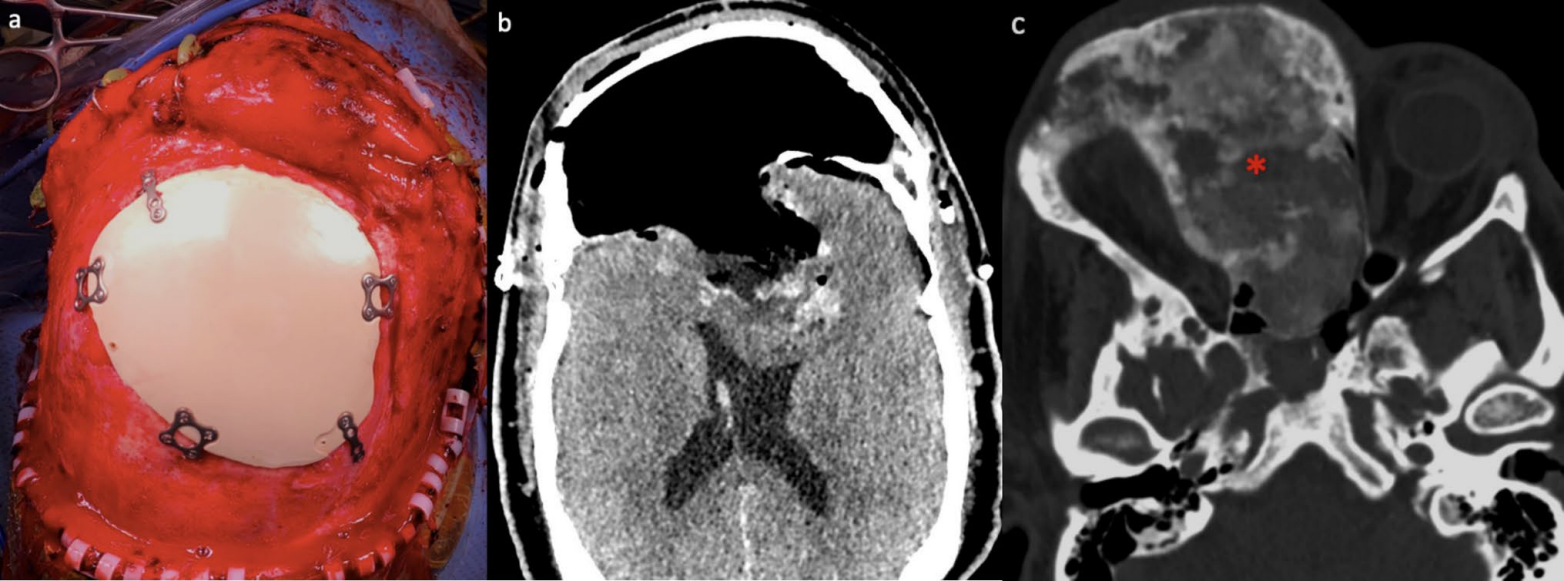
Second surgery images. a Intraoperative picture demonstrated the placement of the custom-made PEEK cranioplasty, fixed with multiple titanium miniplates, perfectly fitting the cranial defect. b Axial CT scan after the second surgery showed the removal of the extensive FD lesion, brain decompression, and reconstruction of the anterior calvarium. c Axial CT scan with bone settings window highlighted the residual disease
